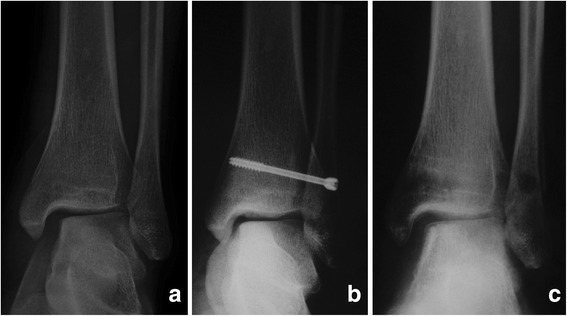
a An AO/OTA type-C ankle fracture with enlarged medial clear space and syndesmotic space. b The patient was fixed with a syndesmotic screw, and the medial clear space was reduced to normal. c One year postoperative X-ray showed malreduction of the medial clear space although without symptoms
